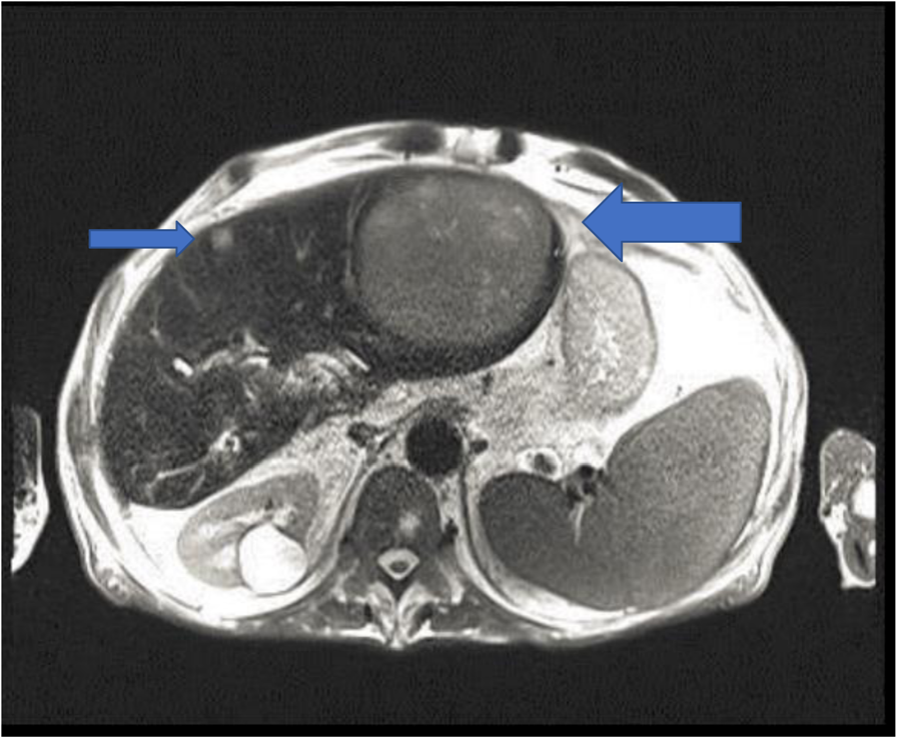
MRI of the liver as described in Fig. 2; the size of the subcapsular nodule (smaller arrow) has not increased compared to that of Fig. 1. The supposed third nodule (Fig. 4) was not confirmed
